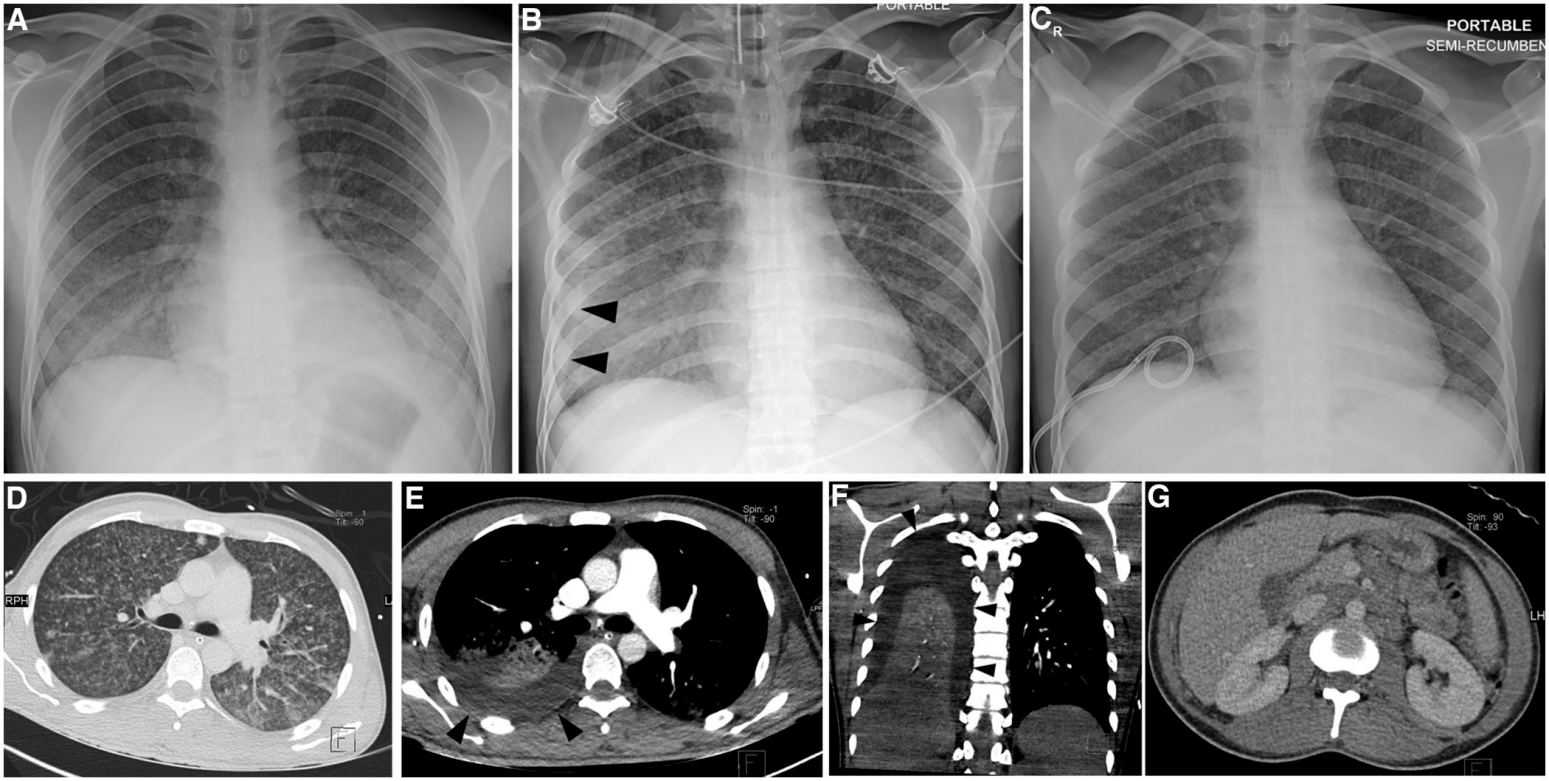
Development of pleural effusion in 24-year-old man with miliary TB. (A) Initial frontal chest radiograph shows multiple tiny nodules in both lung fields, typical of miliary TB. (B) Portable chest radiograph taken 7 days later after the patient’s condition deteriorated shows slight blunting of the right costophrenic angle and right peripheral pleural opacity (black arrowheads). (C) Repeat chest radiograph after drainage catheter insertion shows resolution of right pleural effusion. (D) Initial axial CT image taken in the lung window shows miliary nodules in both lung fields. (E) Corresponding axial and (F) coronal CT images taken 5 days later show right pleural effusion (black arrowheads) and underlying lung atelectasis. (G) Axial contrast-enhanced CT image of the abdomen shows extensive paraaortic, peripancreatic and superior mesenteric lymphadenopathy.
